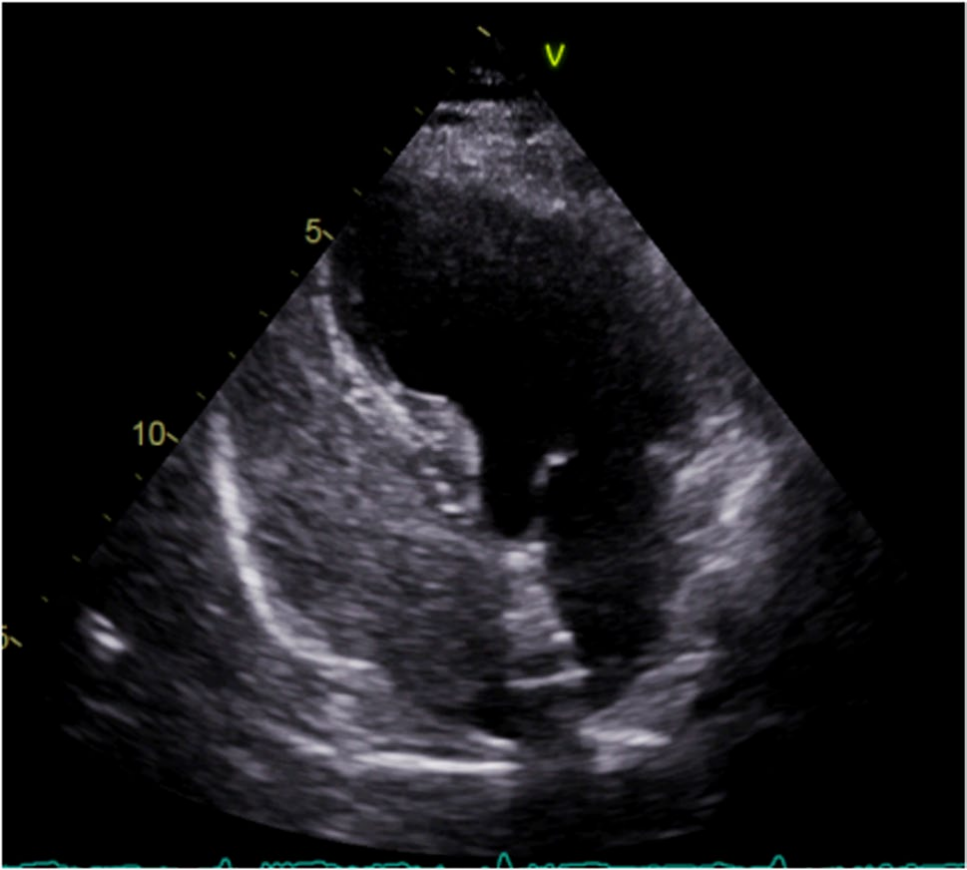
The patient`s the latest right heart contrast echocardiography showed no microbubbles
